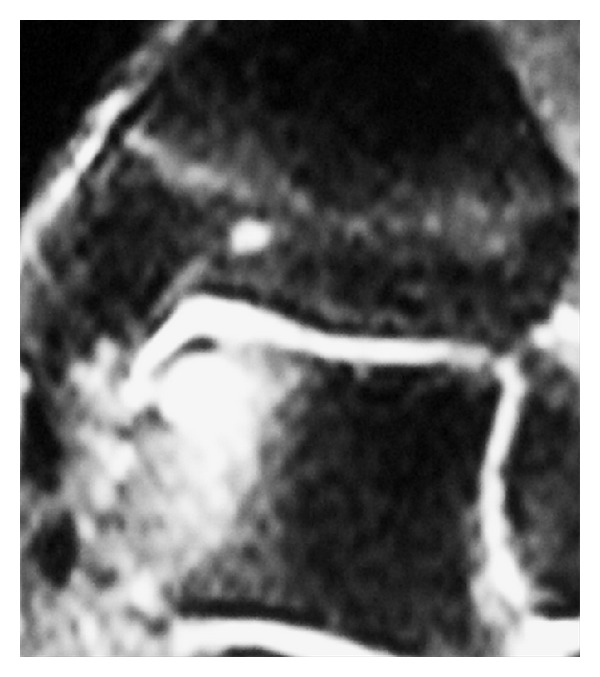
Coronal MRI view 2 years after the first operation. The cartilaginous layer was present, but it was submined by a subchondral cyst.
